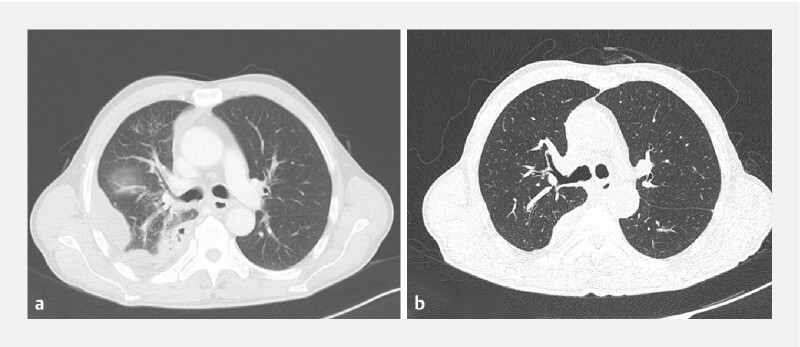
Computed tomography scan.
